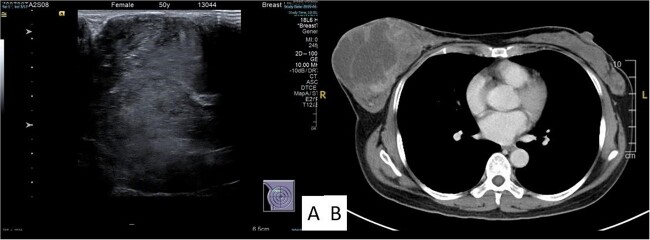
Ultrasound (A) and computed tomography (B) image of the tumor.
